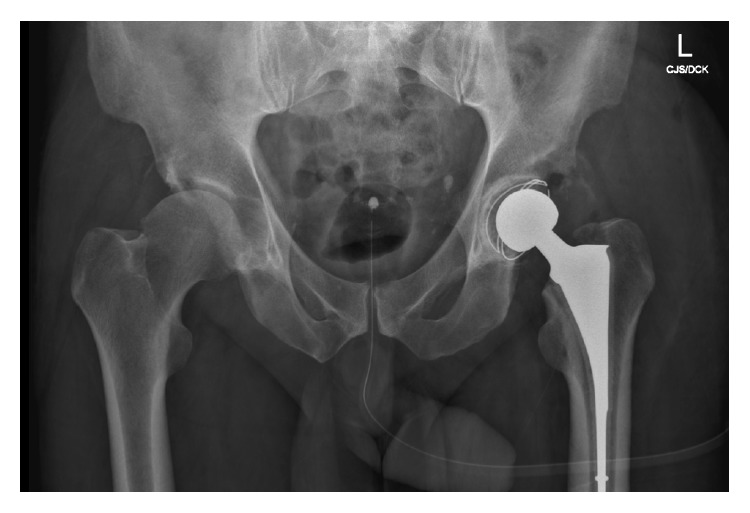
Postoperative radiograph demonstrating first-stage cemented total hip replacement with 48/32 mm Stryker RimFit Acetabular cup and size 9 Biomet Simplex cement spacer.
